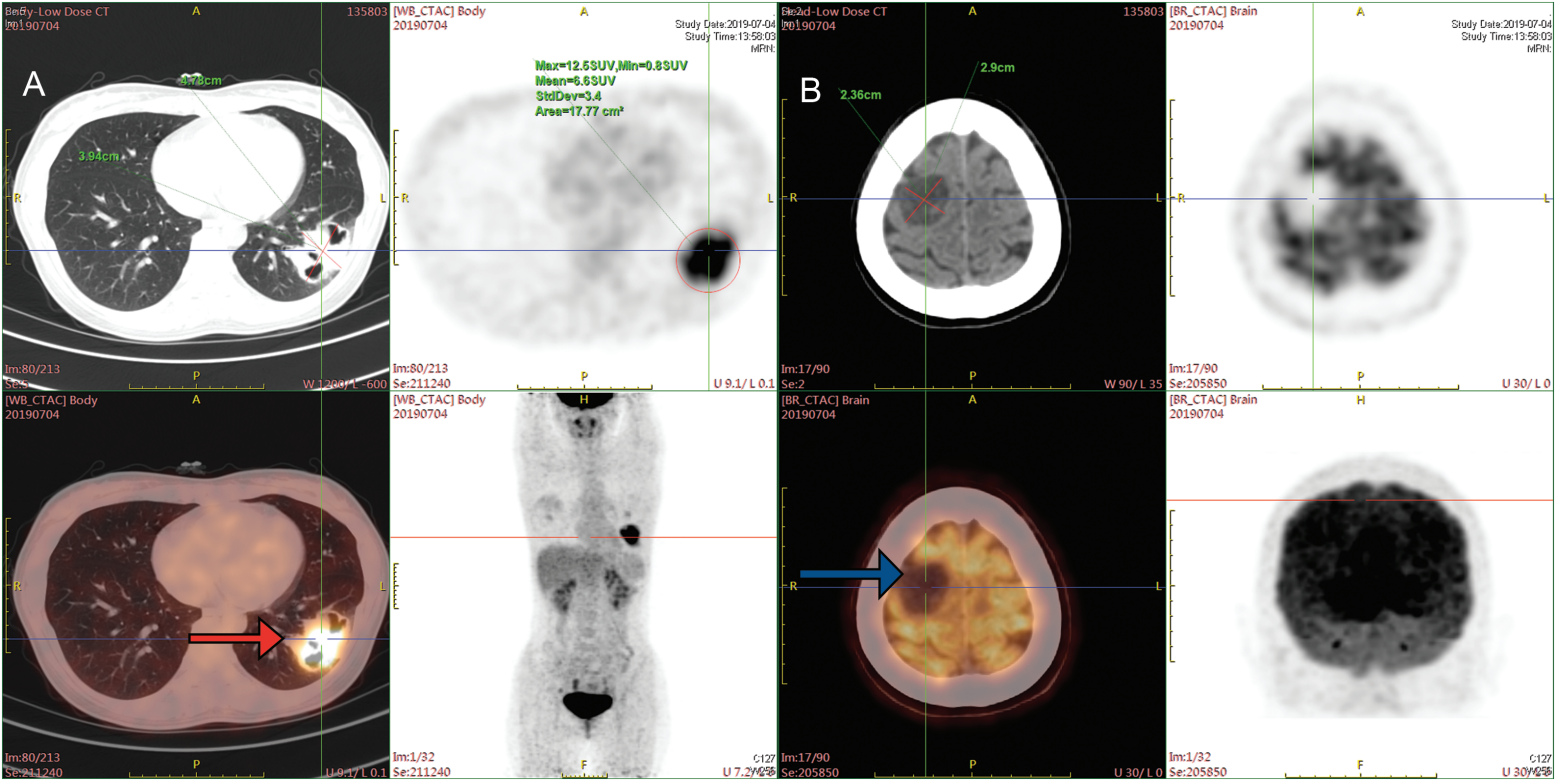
PET-CT images at the time of initial diagnosis. (A) PET-CT on 04 July 2019, before treatment. The mass at the peripheral basal segment of the left lower lobe showed increased FDG uptake (red arrow). (B) Low-density lesions in the right parietal lobe, with increased FDG metabolism (blue arrow). PET-CT = Positron emission tomography-computed tomography, FDG = fluorodeoxyglucose
